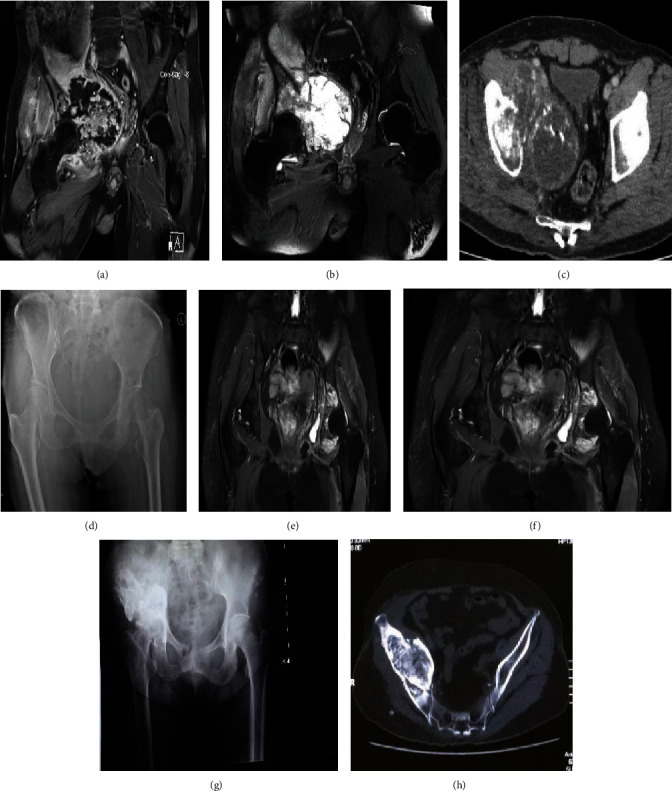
Preoperative radiography, MRI, and CT images. (a) Coronal T2-weighted perfusion MRI scan demonstrating tumor involvement in pelvic regions I+II+III. (b) Coronal T2-weighted MRI scan demonstrating tumor involvement in pelvic regions I+II+III. (c) CT image demonstrating tumor involvement in pelvic regions I+II+III. (d) X-ray image demonstrating tumor involvement in pelvic regions II+III. (e) Coronal T2-weighted perfusion MRI scan demonstrating tumor involvement in pelvic regions II+III. (f) Coronal T2-weighted MRI scan demonstrating tumor involvement in pelvic regions II+III. (g) X-ray image demonstrating tumor involvement in pelvic regions I+II. (h) CT image demonstrating tumor involvement in pelvic regions I+II.
